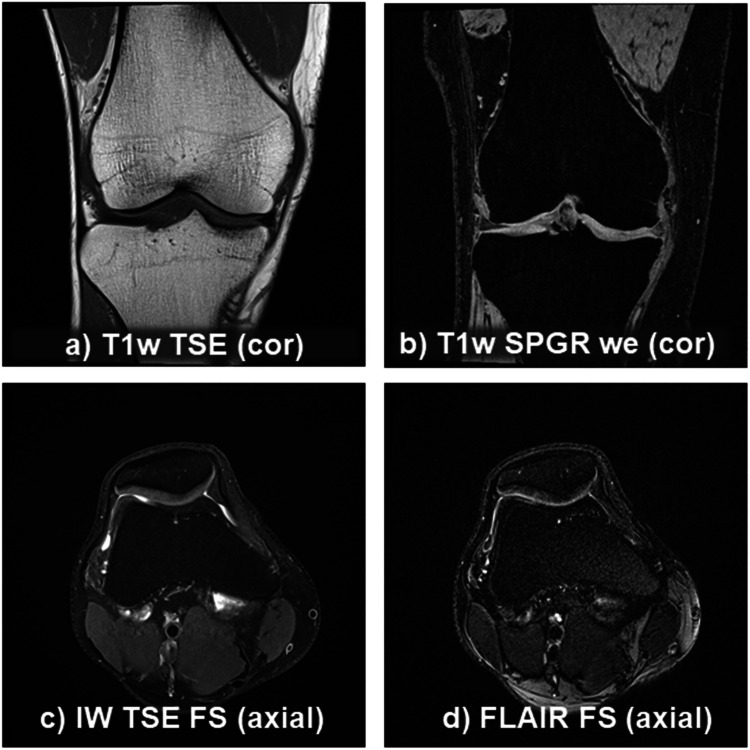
Multifaceted MRI with different contrasts commonly used in osteoarthritis imaging, visualizing a the general anatomy, collateral ligaments, bone pathology, etc.; b cartilage morphometry (thickness, volume, surface, and denuded areas, etc.); c effusion synovitis, femora-patellar cartilage pathology, bone marrow lesions (BMLs), etc.; and d separation of thickened synovium and effusion
